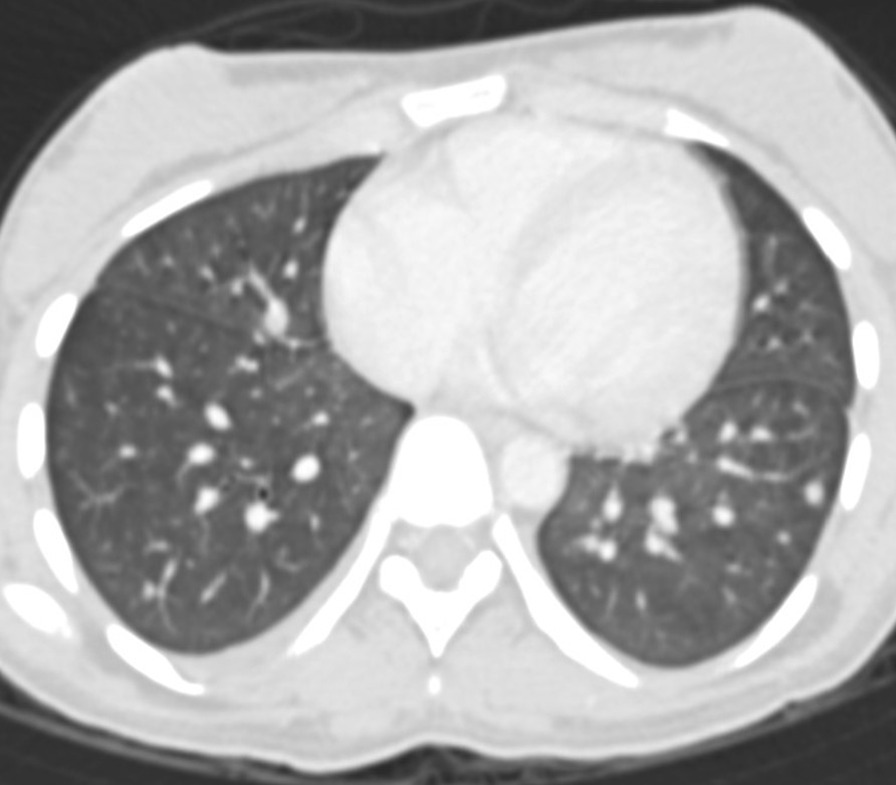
Follow-up axial computed tomography scan showing a significant reduction of pulmonary nodules and ground-glass changes in the middle lobe, lingular segment of left upper lobe, and bilateral lower lobes
